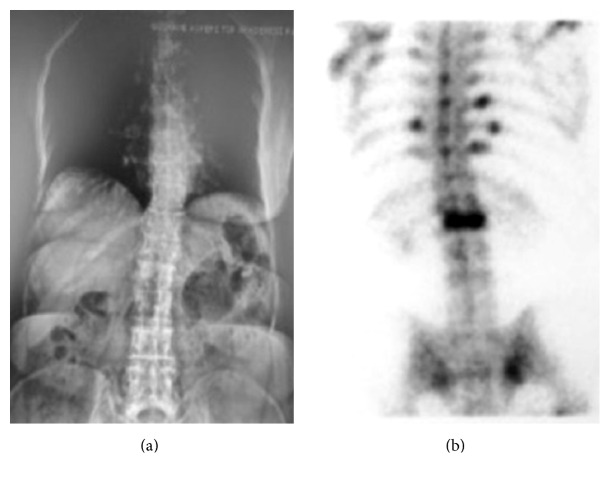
(a) Plane radiography and (b) scintigraphy showing L1 osteoporotic vertebra fracture.
